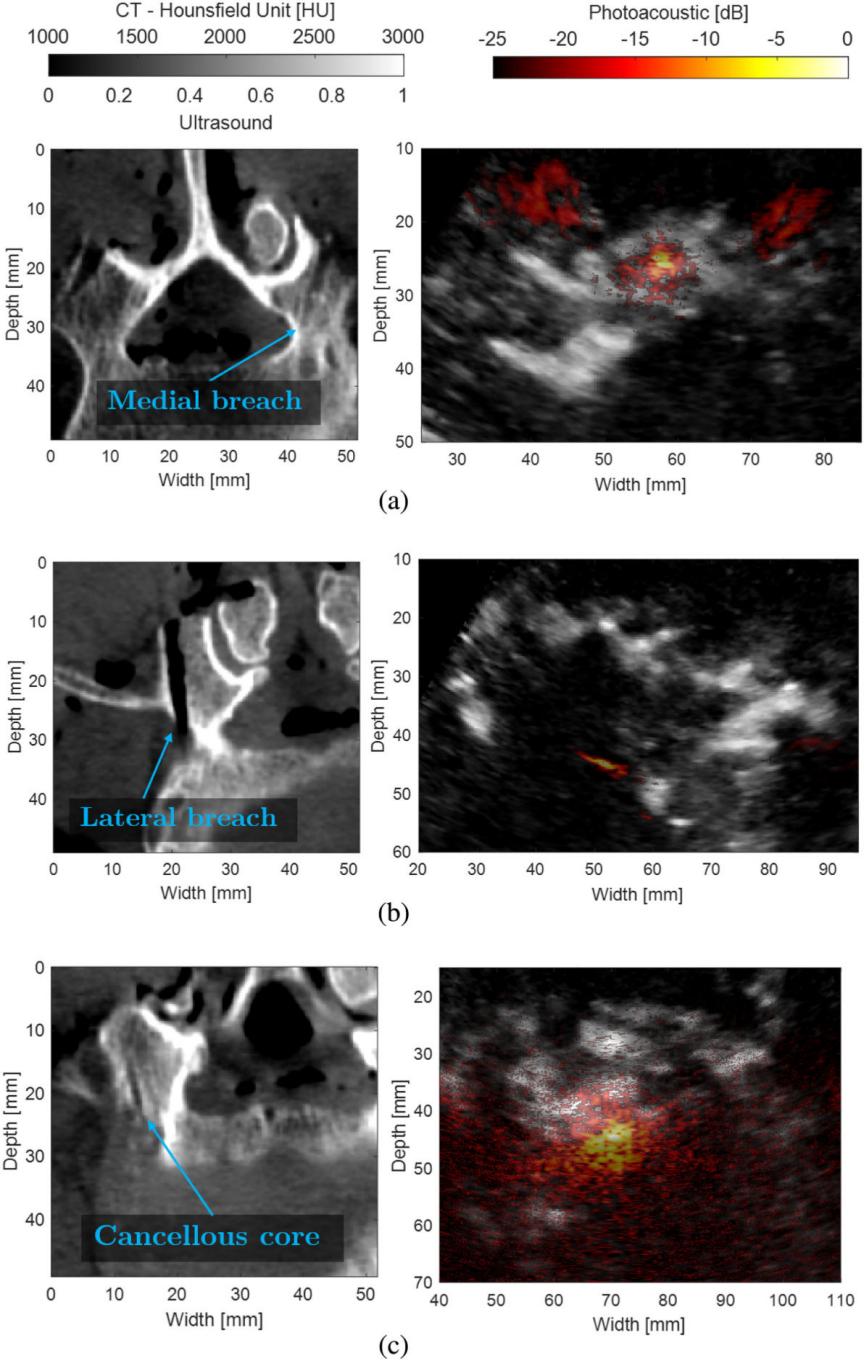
Examples of photoacoustic signals generated when the tip of the optical fiber is touching a (a) medial breach, (b) lateral breach, and (c) cancellous core. Left column: CT axial slice. Right column: LW-SLSC ultrasound image co-registered with DAS photoacoustic image.
